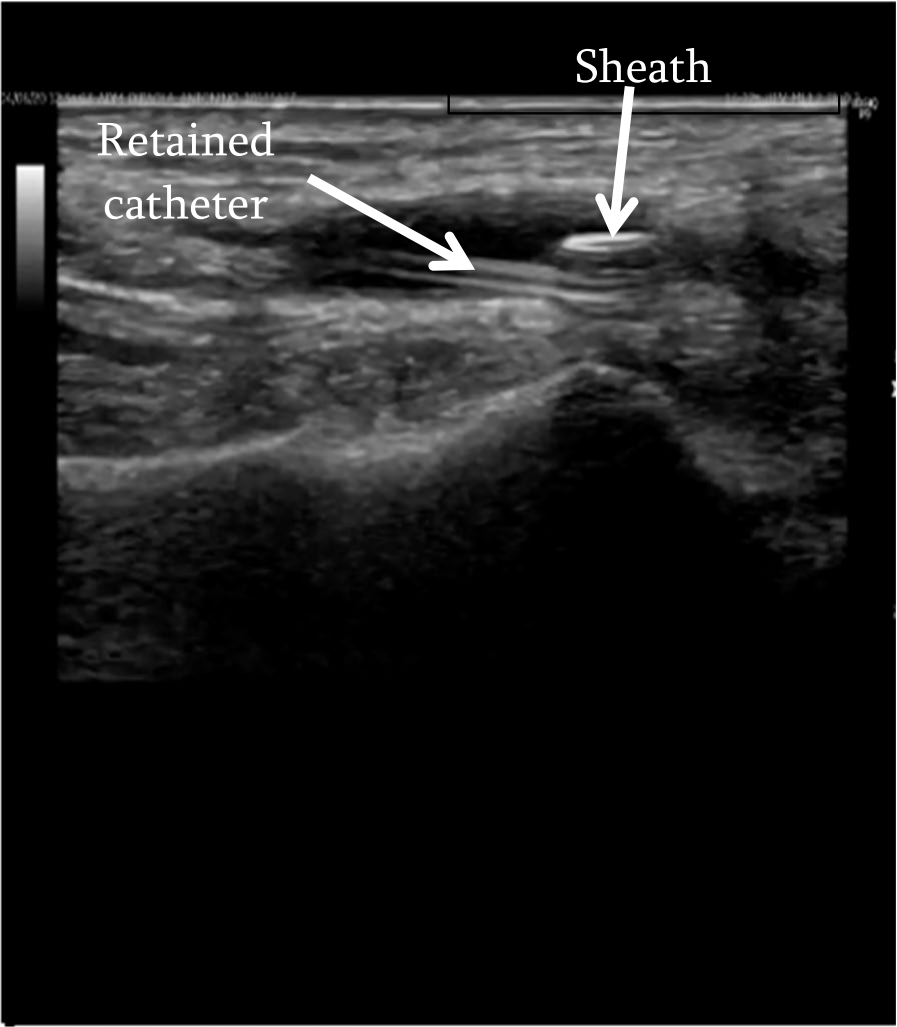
Ultrasound image demonstrating tracking back of retained fragment into sheath
